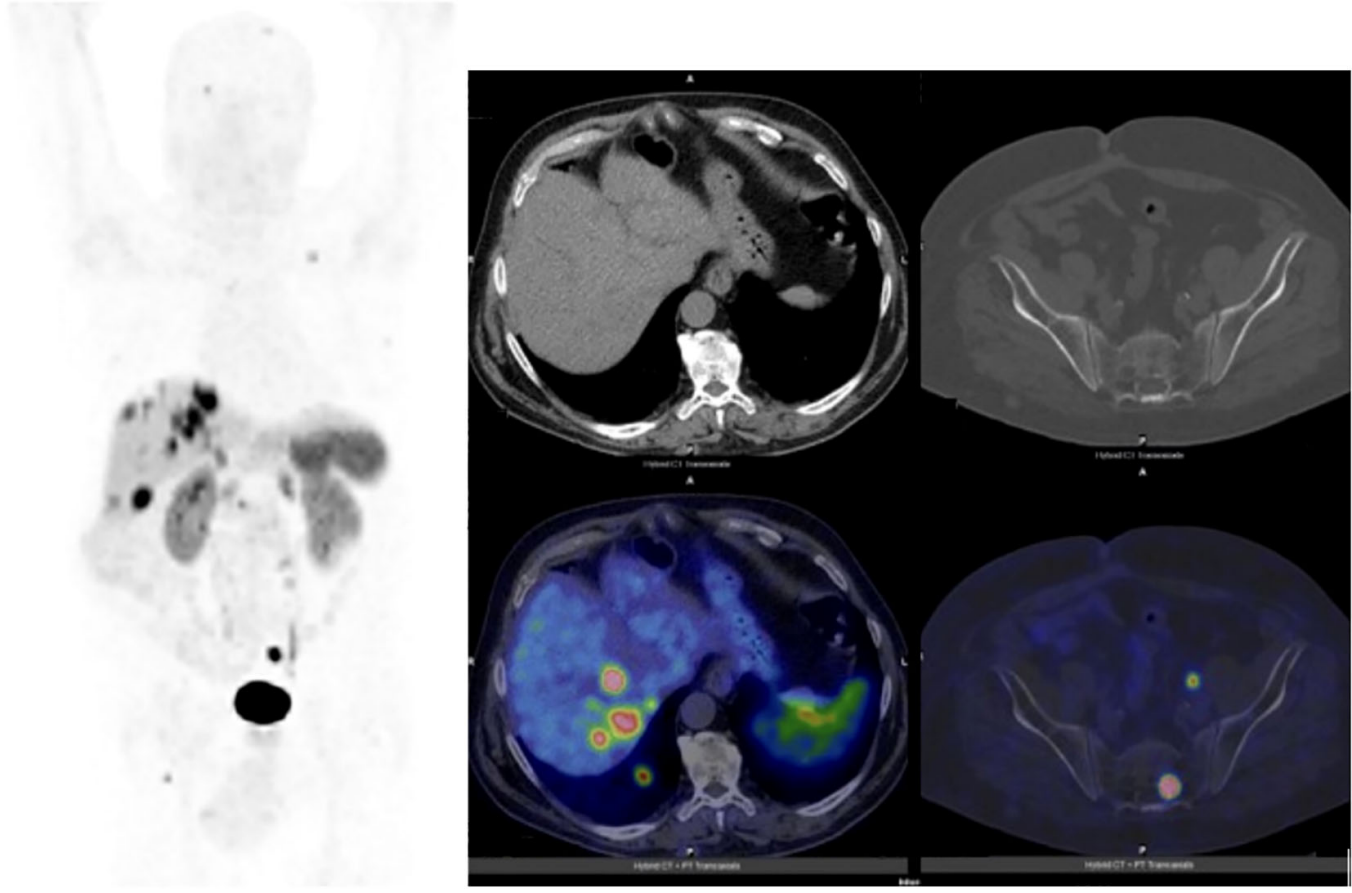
Maximum-intensity-projection and transaxial images of a the [68Ga]Ga-DOTATOC PET/CT performed in March 2023 for restaging after somatostatin analogues initiation. The PET scan demonstrated disease progression due to the appearance of SST uptake in multiple secondary hepatic and bone lesions. 125.
